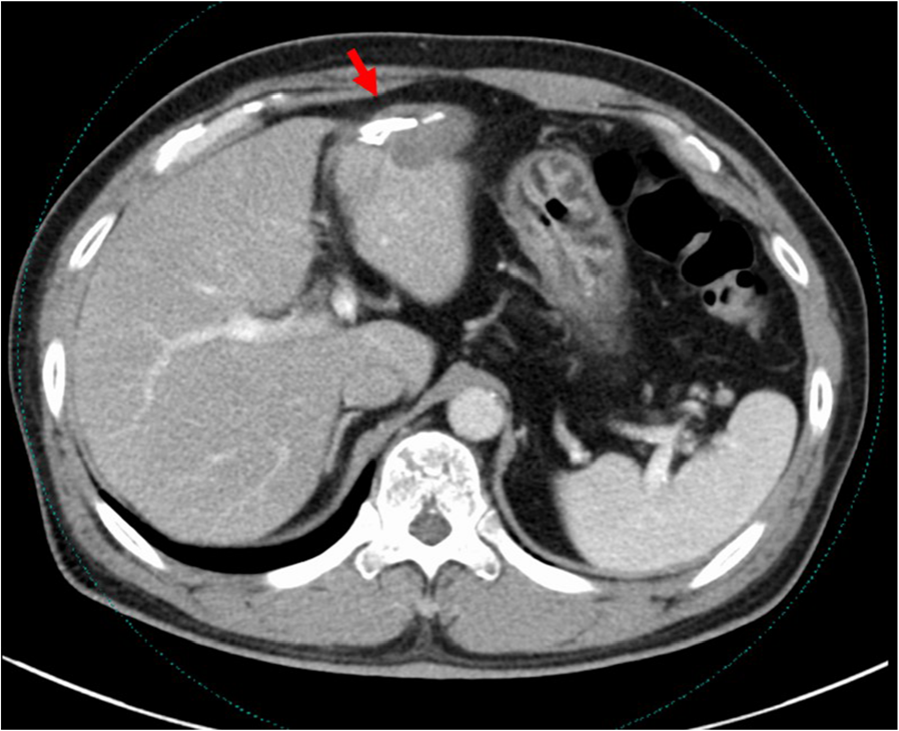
Contrast-enhanced CT scan. A non-enhanced mass (arrow) measuring 35 mm in length at the wedge from the cut stump of the hepatic resection
